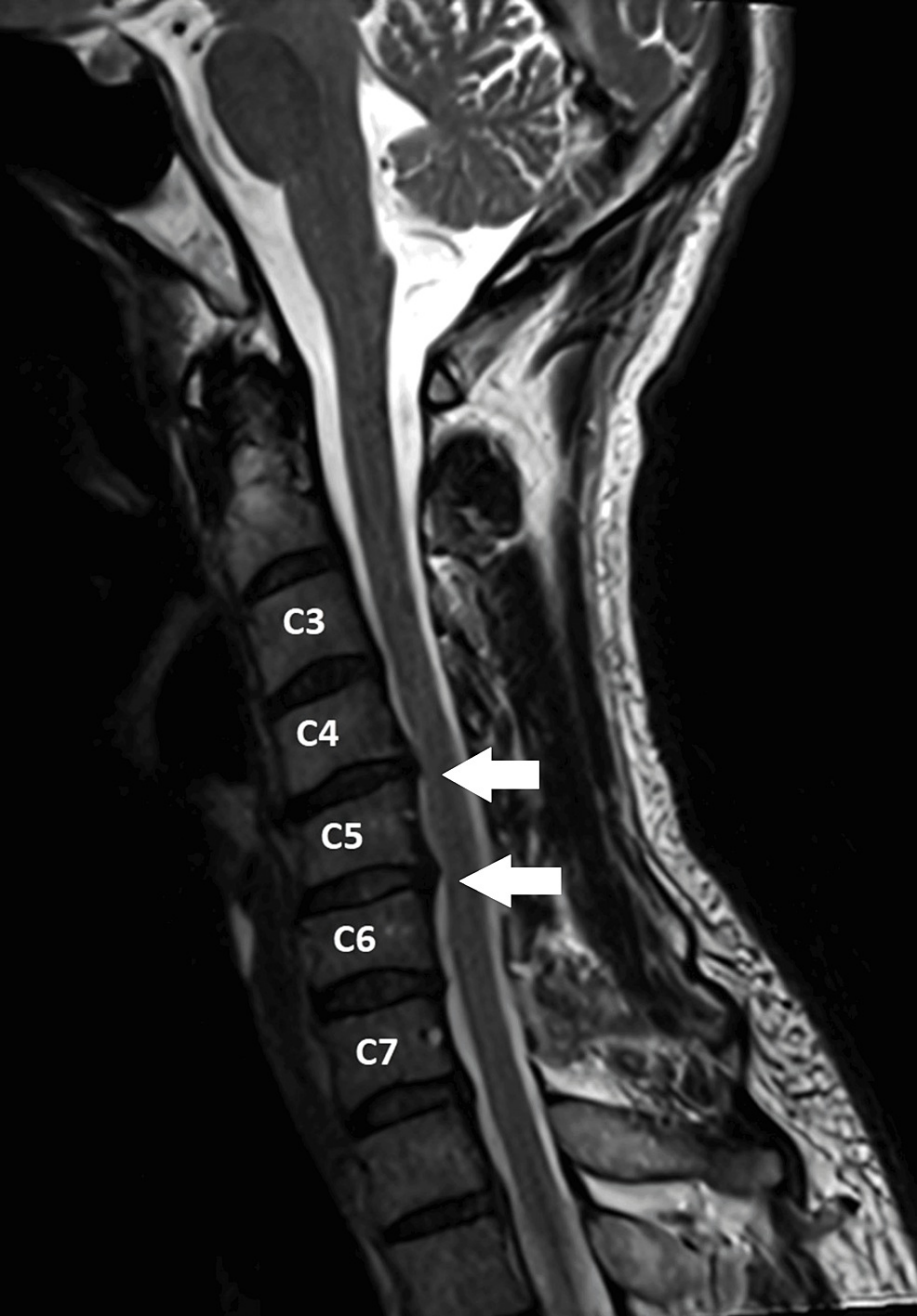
Cervical magnetic resonance image (MRI). Cervical MRI demonstrating multilevel degenerative changes and nerve root impingement. There is posterior disc bulging/prolapse from C2/3 to C7/T1, resulting in narrowing of the bilateral intervertebral foramina from C4/5 to C7/T1. The spinal cord is abutted at C4/5 and C5/C6. The right C6 nerve root impingement corresponds to the patient’s radicular symptoms in the C6 distribution.
